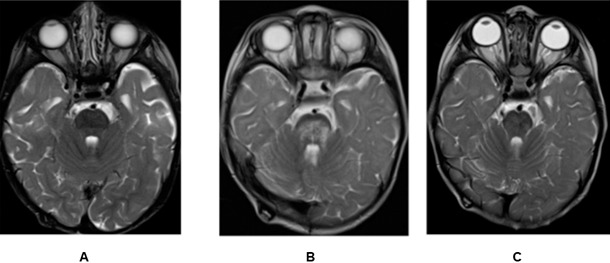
T2-FLAIR MRI images of single patient with CTCAE grade 1 brainstem changes (A) before radiation, (B) 7.5 weeks after radiation (scan demonstrating new onset patchy edema with microhemorrhages in the pons), and (C) 6 months following completion of radiation (MRI demonstrates resolution of brainstem findings). Abbreviations: CTCAE, Common Terminology Criteria for Adverse Events; FLAIR, fluid-attenuated inversion recovery; MRI, magnetic resonance imaging.
